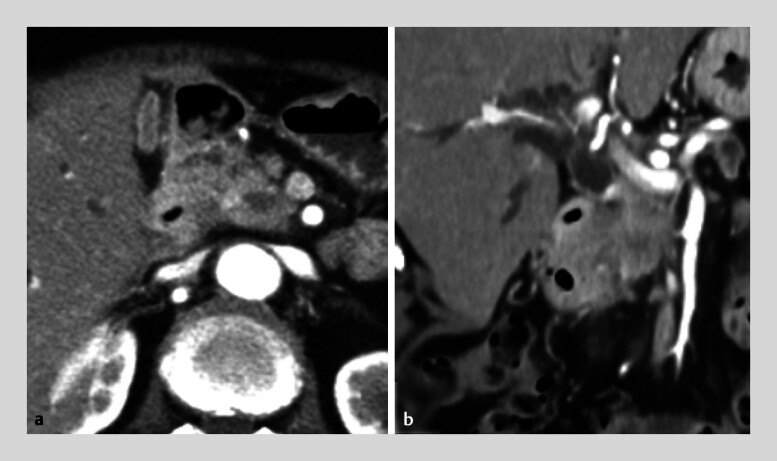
Contrast-enhanced computed tomography scans showing a hypovascular tumor in the pancreatic head.
